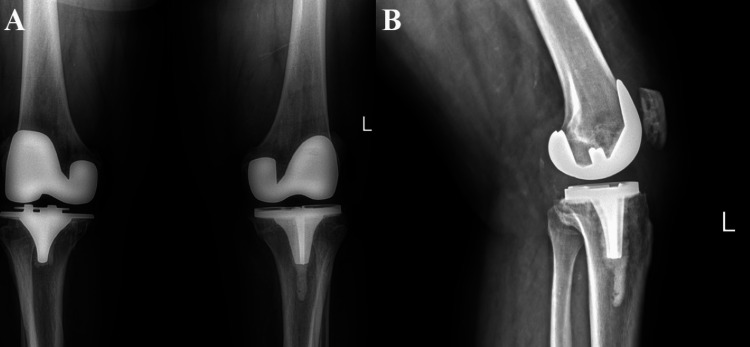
(A) POD-15 anteroposterior demonstrating well-positioned bilateral total knee arthroplasty. (B) POD-15 lateral radiograph demonstrating a well-positioned left total knee arthroplasty. (A) POD-15 anteroposterior X-ray demonstrates a well-positioned total knee arthroplasty bilaterally with stable osseous integration and no signs of loosening. (B) POD-15 lateral X-ray demonstrates a well-positioned total knee arthroplasty in the left knee with stable osseous integration and no signs of loosening. POD, postoperative day
